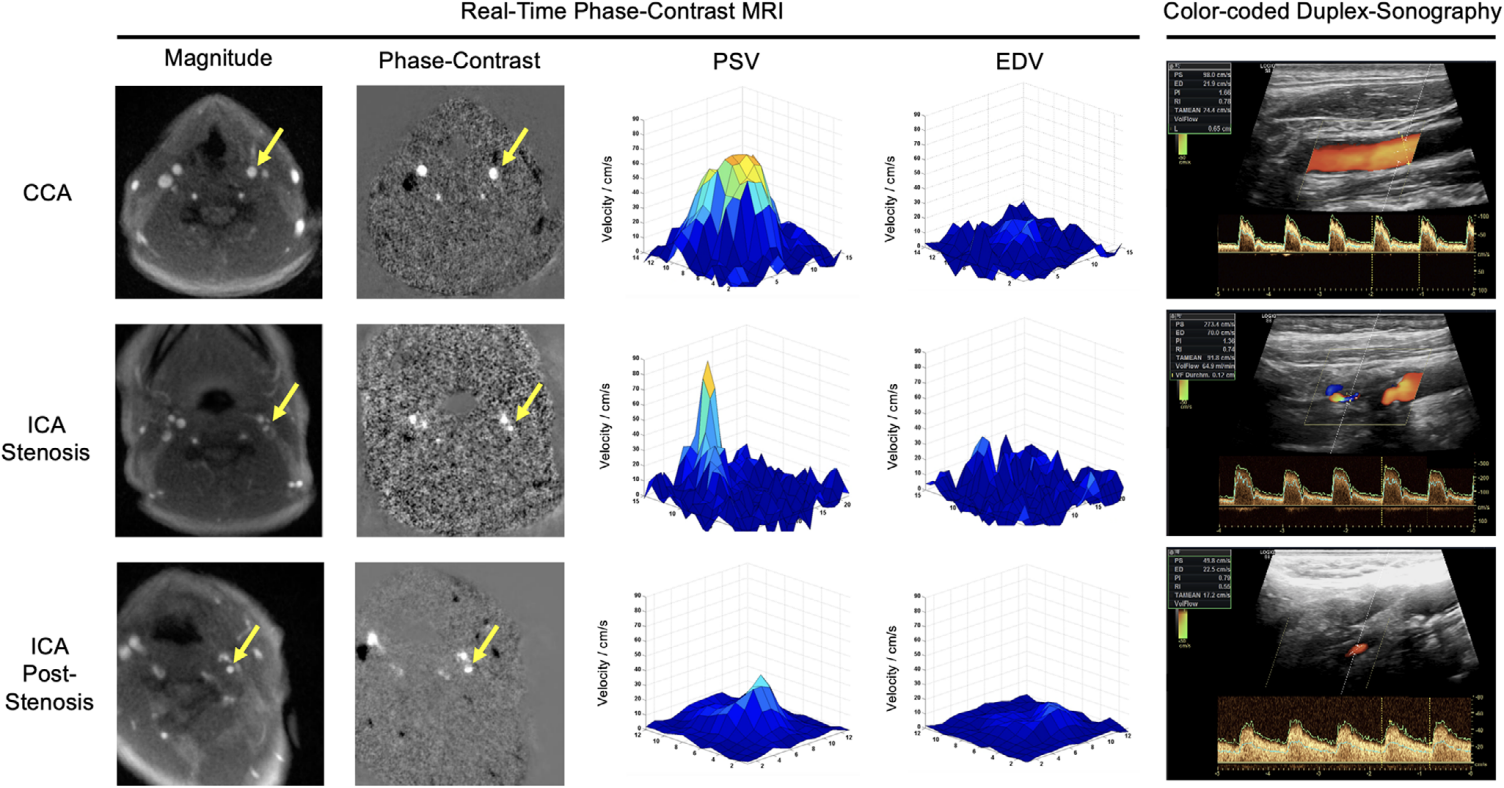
Patient with high‐grade carotid artery stenosis: Representative transversal real‐time phase‐contrast MRI images of the anatomy (magnitude images) and velocity‐coded phase‐contrast images, three‐dimensional representations of the velocities in plane at peak systole (PSV) and end diastole (EDV), as well as representative longitudinal color‐coded duplex sonography images at the common carotid artery (CCA) and internal carotid artery (ICA) at the maximum of the stenosis and post‐stenosis. In the magnitude images, all arteries and veins are hyperintense; in the phase‐contrast images, arteries are hyperintense (upward flow) and veins are hypointense (downward flow). The yellow arrows mark the left CCA, intra‐stenotic ICA, and post‐stenotic ICA. Note the increased pulsatility in the CCA (pre‐stenotic flow pattern) and decreased pulsatility of the distal ICA (post‐stenotic flow pattern). PS, peak systole; ED, end diastole; PI, pulsatility index; RI, resistance index; TAMEAN, time‐averaged mean velocity; Durchm, diameter.
